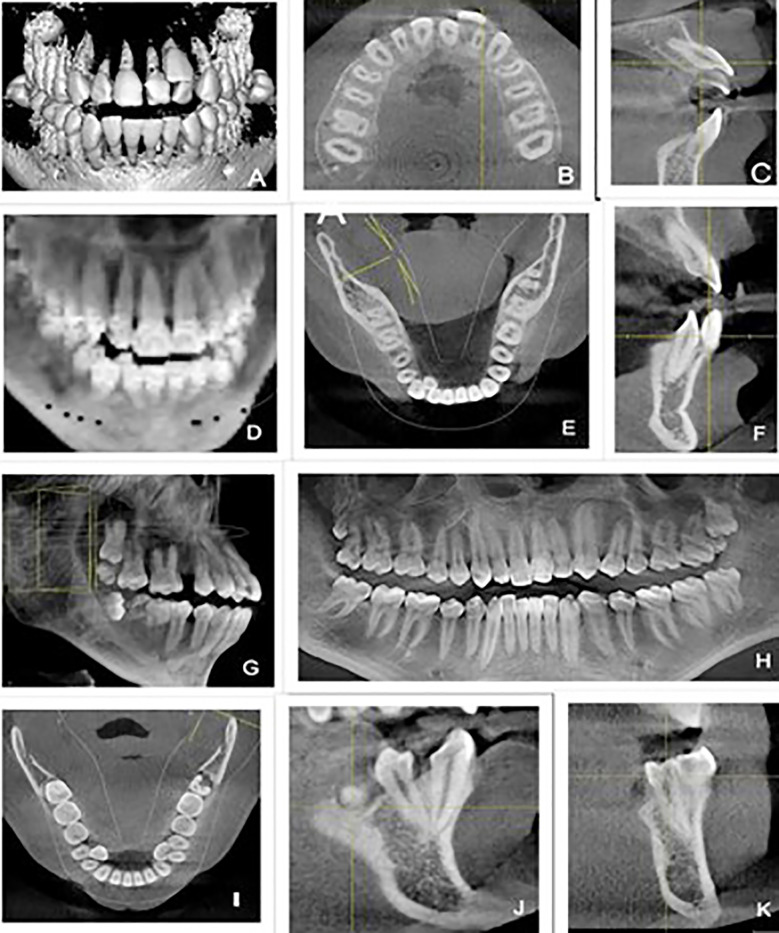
CBCT and Panoramic Views Demonstrating a Range of Supernumerary Teeth with Variations in Number, Location, Morphology, Orientation and Developmental Stages.
